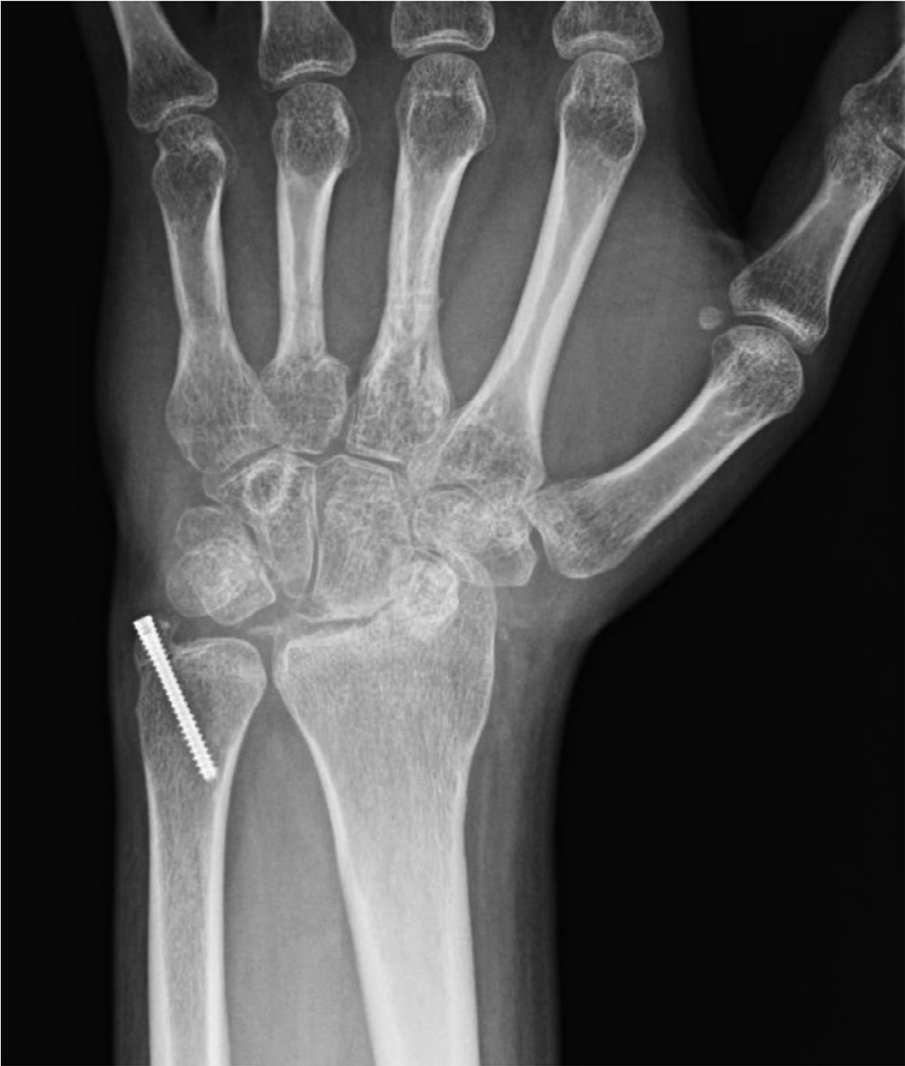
Patient 3: Subsequent radiographs obtained 7 months after the radiographs in Figure 3b show interval removal of hardware, with a headless screw remaining across the ulnar styloid fracture. The proximal pole of the scaphoid and the lunate are no longer visible, compatible with bony resorption/collapse; these bones were not surgically removed. The capitate now articulates with the distal radius with associated degenerative changes. The third and fourth metacarpal base fractures demonstrate interval healing.
